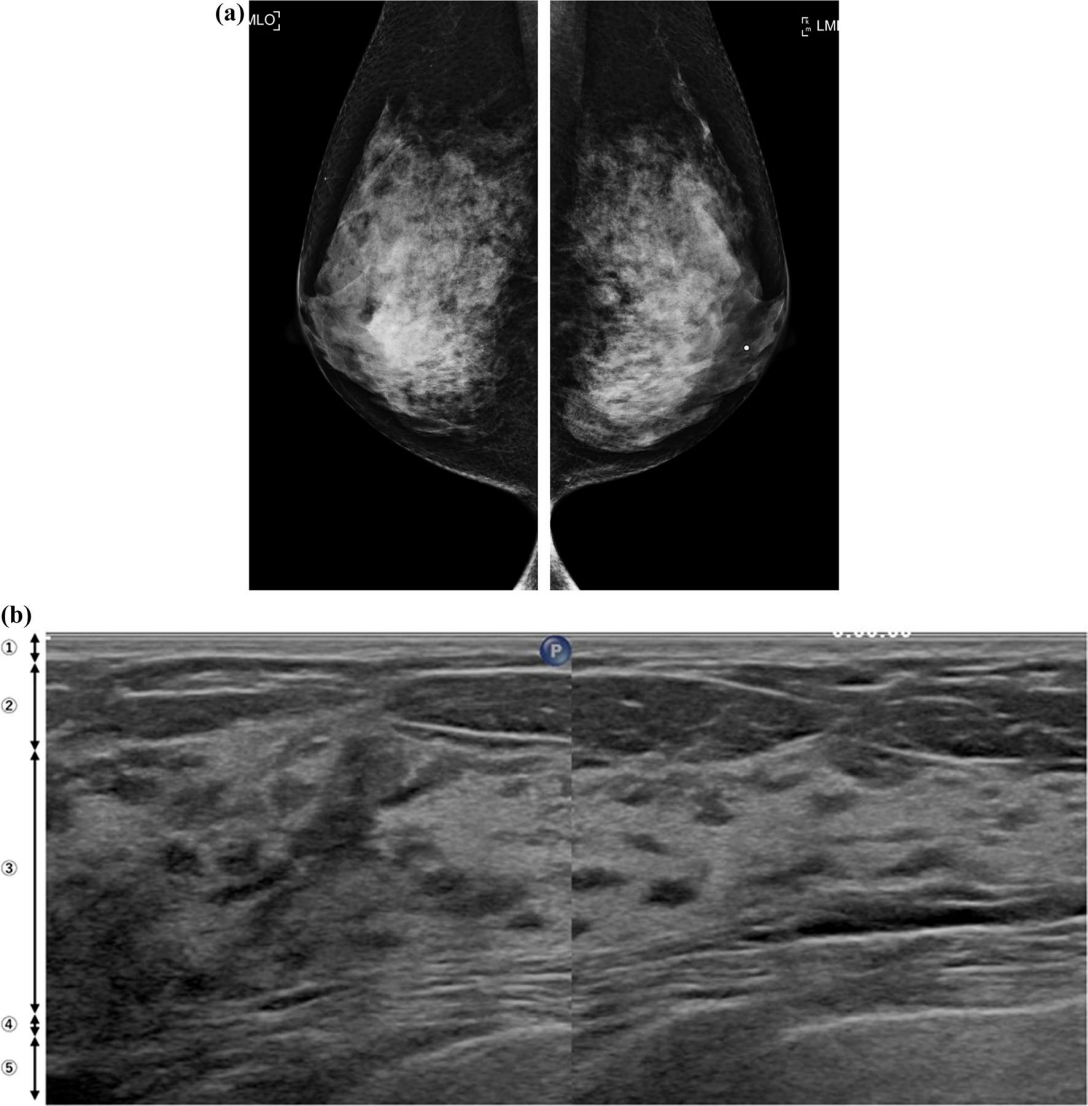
Mammographic dense breasts that are also ultrasonographic dense breasts without a high GTC but with a FGT thickness-to-FAT thickness ratio > 0.93. a Mammograms in bilateral mediolateral oblique view showing extremely dense breasts. b This corresponding ultrasonography image shows a FGT thickness-to-FAT thickness ratio > 0.93 without a high GTC. 1, skin; 2, subcutaneous fat; 3, FGT; 4, retromammary fat; 5, chest muscle
